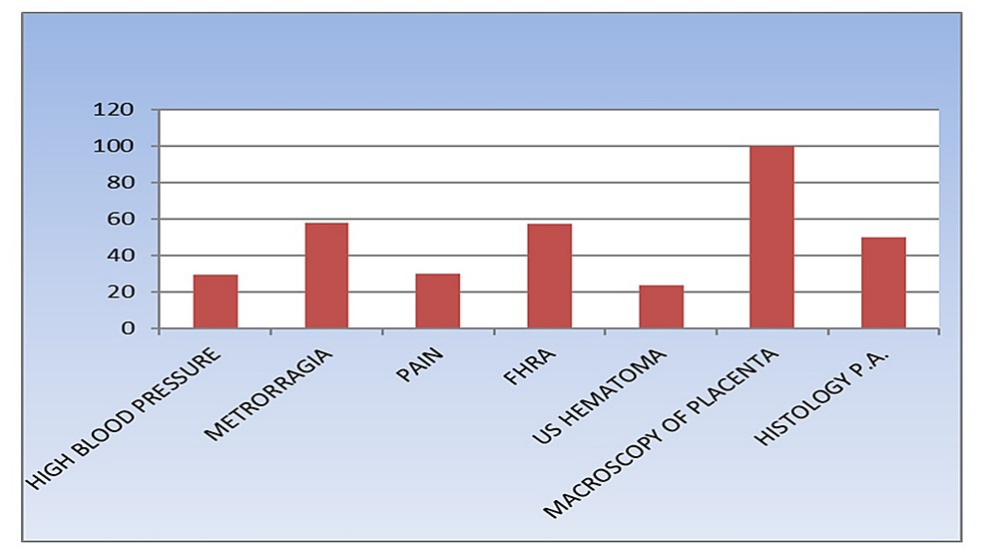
Diagnosis of placenta abruptio (n=201) FHRA: fetal heart rate abnormalities; PA: placental abruption; US: ultrasonography.
